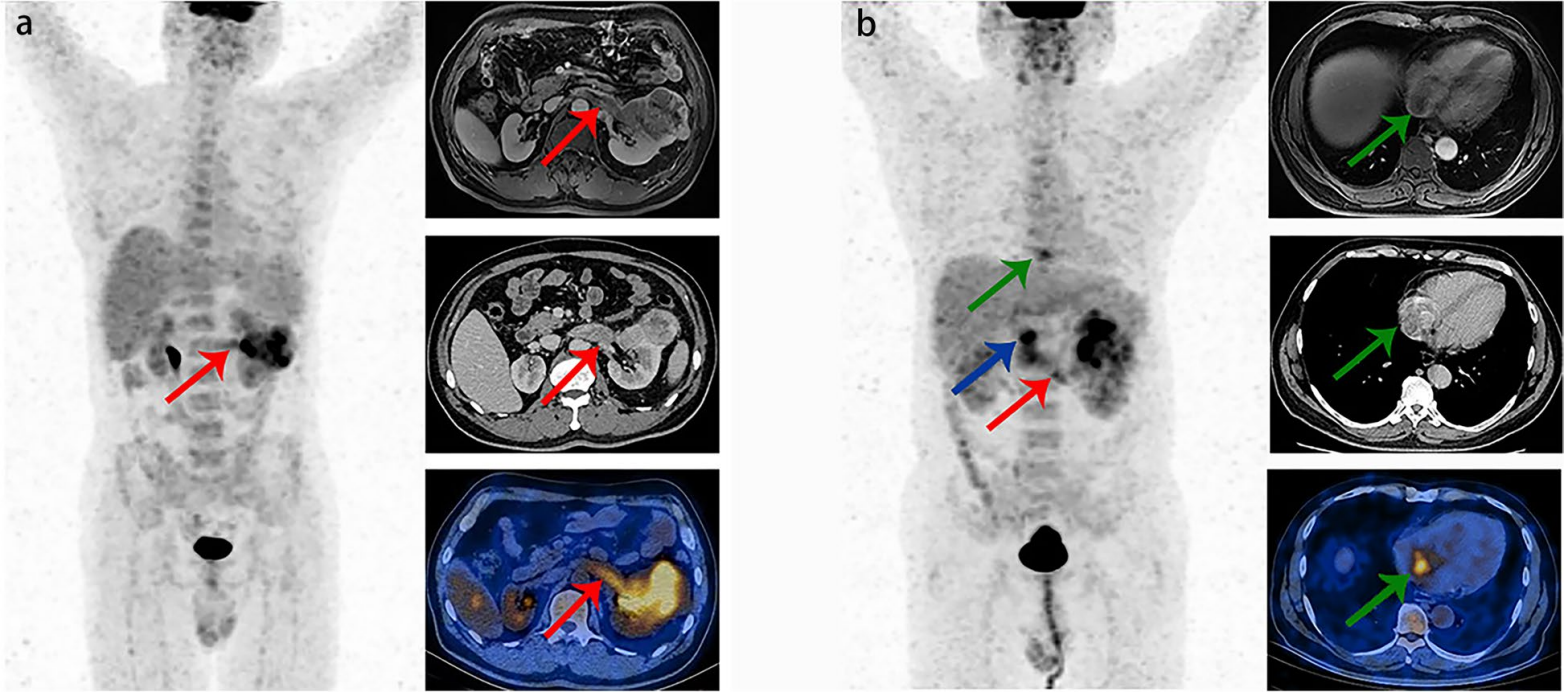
a Images in a 57-year-old male with ccRCC demonstrate left renal vein tumor thrombus (red arrows), with VTT SUVmax of 4.5 (left image: anterior maximum intensity projection image obtained at ^18^F-FDG PET; right upper image: axial contrast-enhanced MRI image; right middle image: axial contrast-enhanced CT image; right lower image: axial fused PET/CT image). b Images in a 55 year-old male with ccRCC demonstrate left renal vein tumor thrombus (red arrow), inferior vena cava tumor thrombus (blue arrow), and tumor thrombus in the right atrium (green arrows), with VTT SUVmax of 5.7 (left image: anterior maximum intensity projection image obtained at ^18^F-FDG PET; right upper image: axial contrast-enhanced MRI image; right middle image: axial contrast-enhanced CT image; right lower image: axial fused PET/CT image)
